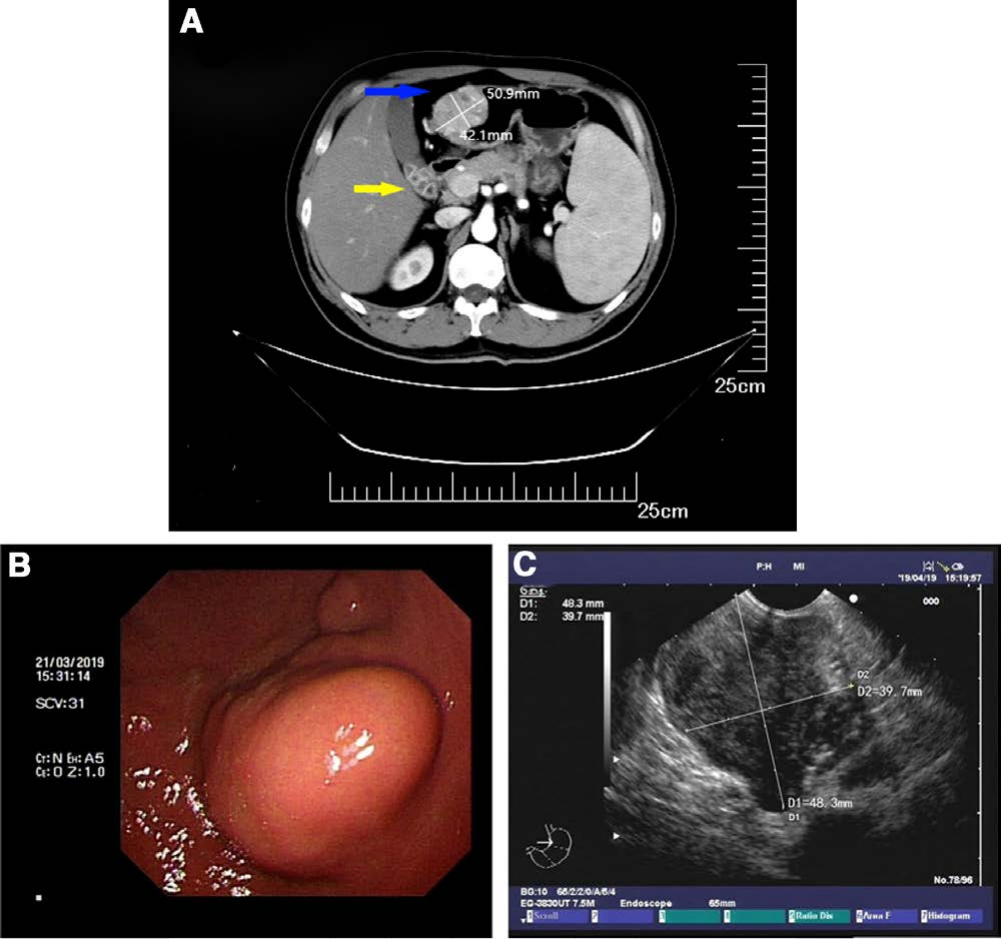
(A) Abdominal CT indicated a 50.9 mm × 42.1 mm mass in the anterior wall of the gastric antrum (blue arrow), with persistent heterogeneous enhancement in the arterial phase and multiple stones in the gallbladder (yellow arrow). (B, C) Ultrasound gastroscopy suggested a 48.3 mm × 39.7 mm hypoechoic mass in the intrinsic muscular layer of the gastric antrum.
